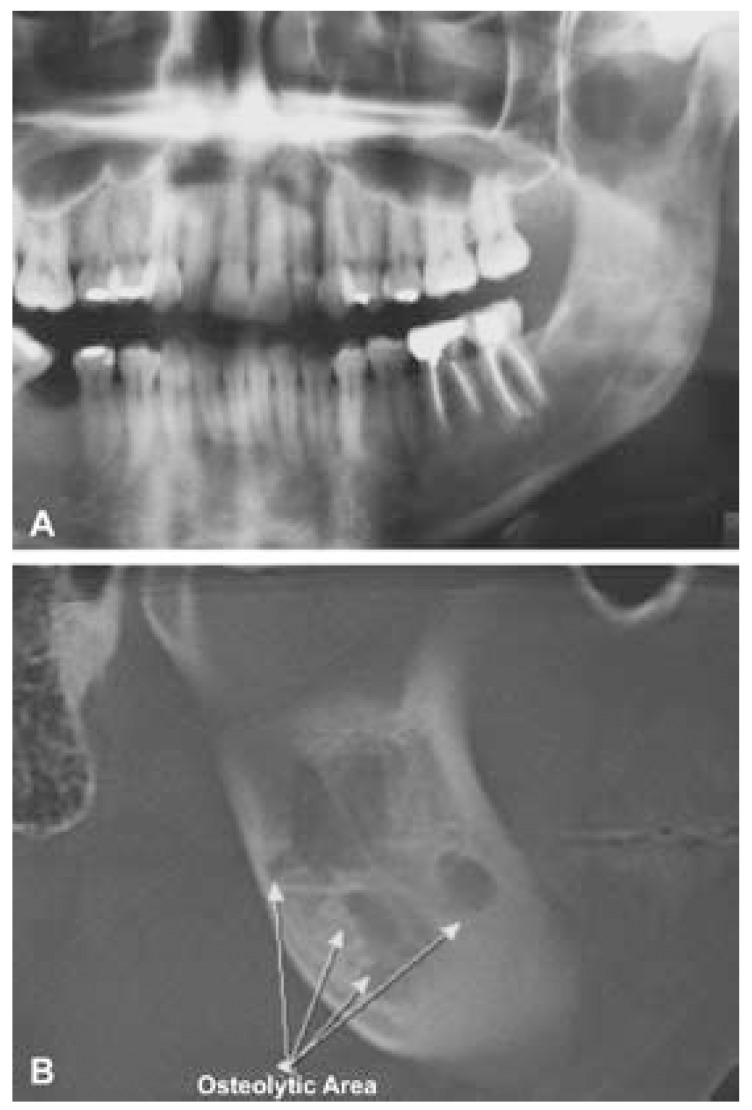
A. Panoramic radiographic images showing an osteolytic ill-defined lesion on the left side of the mandible, extending from the second premolar to the mandibular ramus, and; B. Cone beam CT scan in section images showing osteolytic lesion and destruction of the mandibular vestibular bony cortical.
